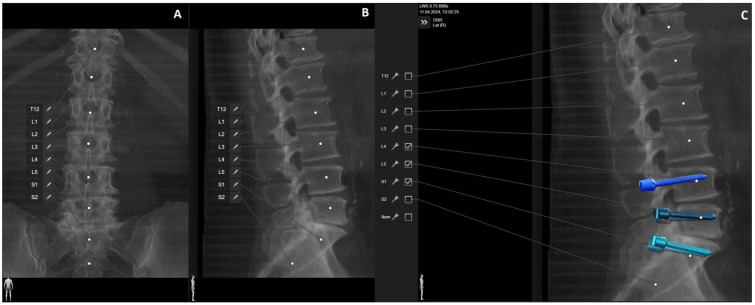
Preoperative (A) anterior–posterior (AP) and (B) sagittal reconstruction of bone anatomy according to preoperative CT with automatic identification of vertebras. (C) Screw recommendations in selected vertebrae for stabilization of L4-S1.
